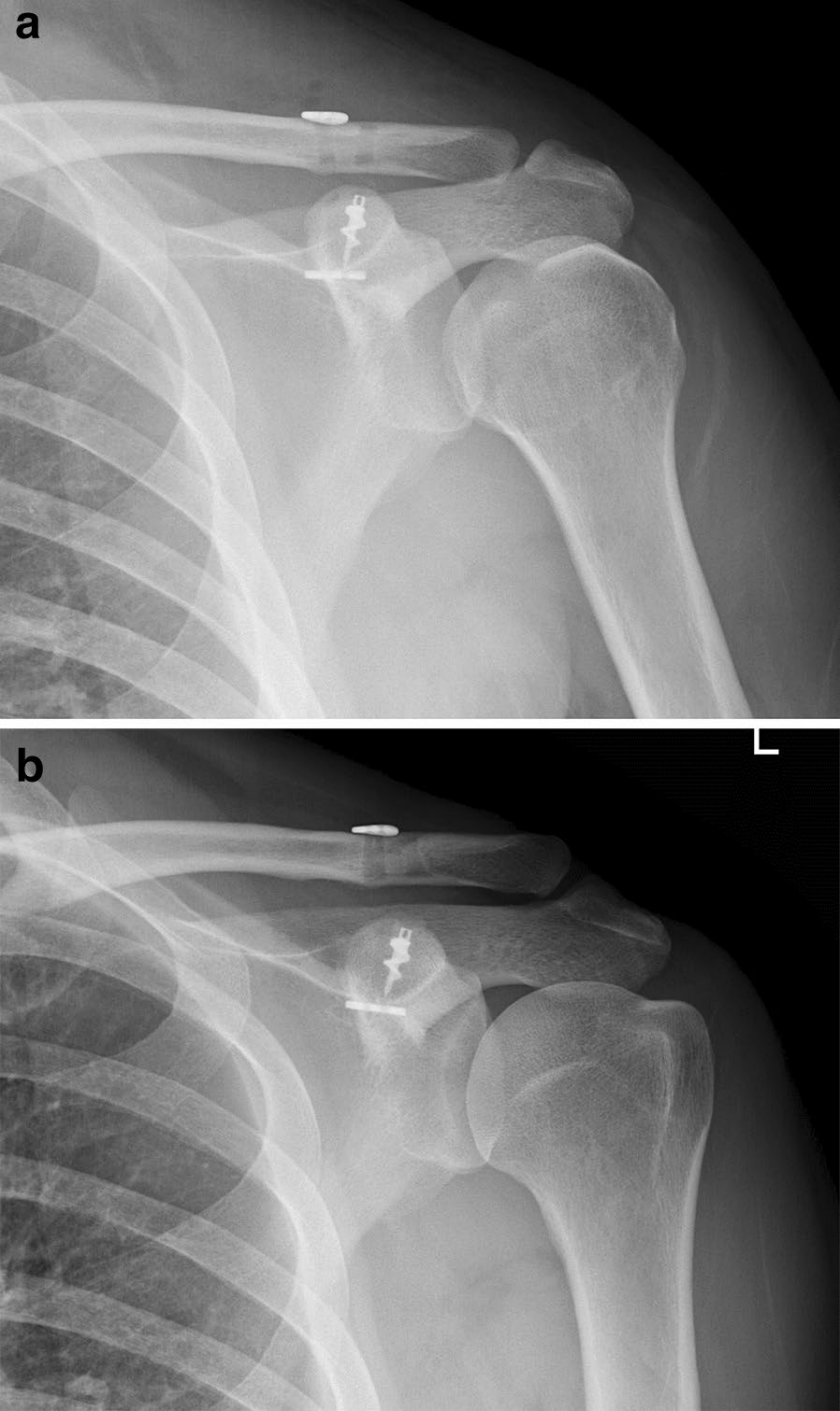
a Successful reduction was achieved with an anchor and a double flip-button although immediate postoperative radiograph revealed slight overcorrection of acromioclavicular joint dislocation. b Subtle reduction loss was observed at 49-months follow-up
